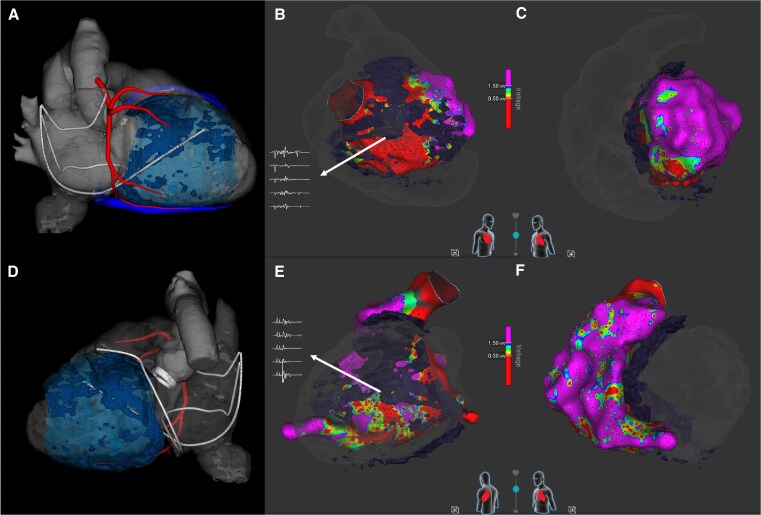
(*A* and *D*) InHEART® images derived from a cardiac computed tomography scan, where scar was defined with delayed contrast enhancement, which is depicted in light and dark blue depending on the density of the scar. (*A*) The left ventricular septum from a right anterior oblique view and (*D*) the right ventricular septum from a left posterior oblique view. The right coronary artery is shown in red, the coronary venous system in blue, and the defibrillator leads in white. The other panels show electroanatomical maps, where colour coding corresponds to voltage. Red represents low voltage (<0.50 mV) and purple represents normal voltage (>1.50 mV). An overlay of dense scar from the scan is shown in dark grey. (*B* and *E*) The left and right ventricles from the same angle as (*A* and *D*), respectively. Local bipolar electrograms in septal dense scar are shown during right ventricular apical pacing. All of the displayed electrograms are pathological and compatible with local abnormal ventricular activation. (*C* and *F*) A left anterior oblique view of both ventricles, respectively.
